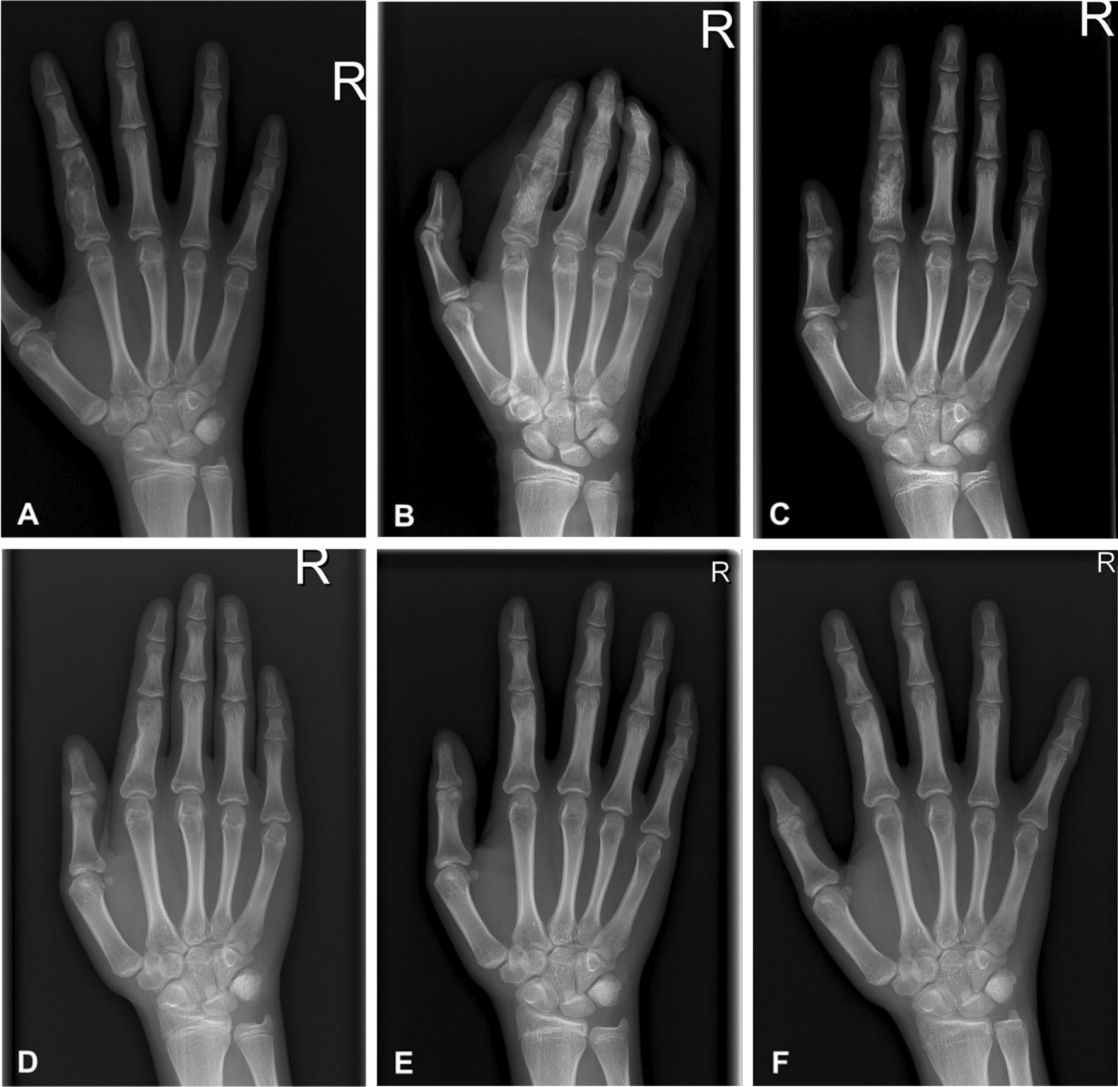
A 13-year-old female with enchondroma of polycentric and giant form in the proximal phalanx of the right index finger. Simple radiographs taken before surgery (A), immediately (B), 1 month (C), 2 months (D), 1 year (E), and 9 years (F) after surgery.
